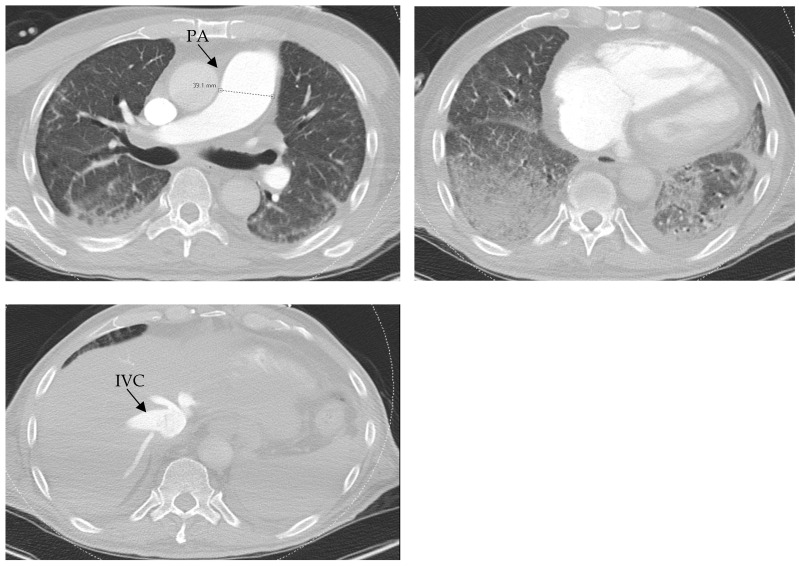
Signs in chest computed tomography (CT) of the lungs associated with pulmonary hypertension (PH). Representative images from a 58-year-old patient with Sjogren’s syndrome complicated by pulmonary hypertension associated with interstitial lung disease (PH-ILD). Upper panel—left: increased diameter of the pulmonary artery (PA, 39 mm), right: right atrial dilation and increased right ventricle to left ventricle diameter. Lower panel: reflux contrast into the inferior vena cava (IVC).
